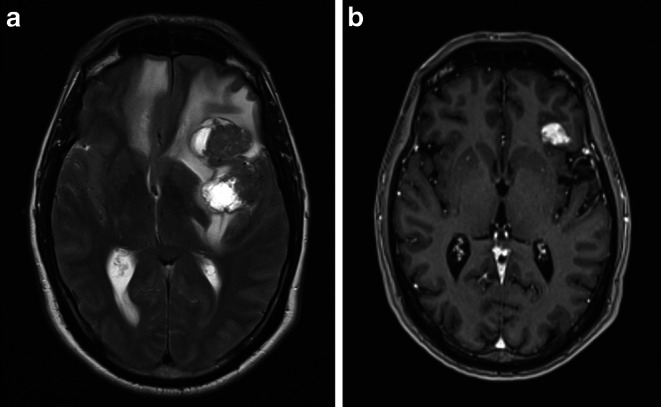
a Brain MRI (fluid-attenuated inversion recovery, FLAIR) of patient 6 in August 2015 before the onset of treatment. Several large brain metastases with perifocal FLAIR hyperintensities in the frontal and parietal lobe, the largest left frontal metastasis measuring 22 × 34 mm. Midline shift to the right by 12 mm and compression of the left lateral ventricle and the third ventricle. The patient was admitted to neurological inpatient care due to symptoms of cerebral compression, which were compensated by dexamethasone treatment. b Brain MRI (T1 with contrast enhancement) in August 2020 at the time of neurocognitive function testing. Residual, stable brain metastases after radiotherapy and checkpoint inhibitor treatment with ipilimumab and nivolumab. At this point, the patient had an overall average neurocognitive function, allowing a return to his job as a teacher
